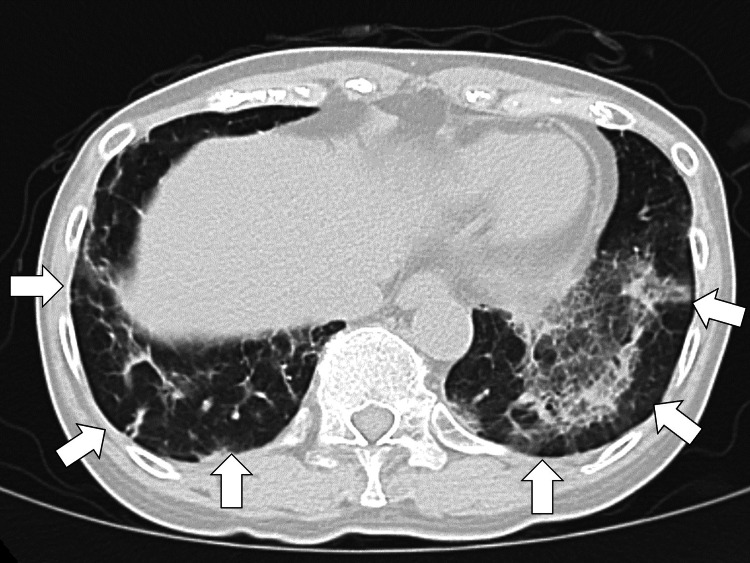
A follow-up chest computed tomography shows an exacerbation of the infiltrative shadows in both lungs (white arrows).
